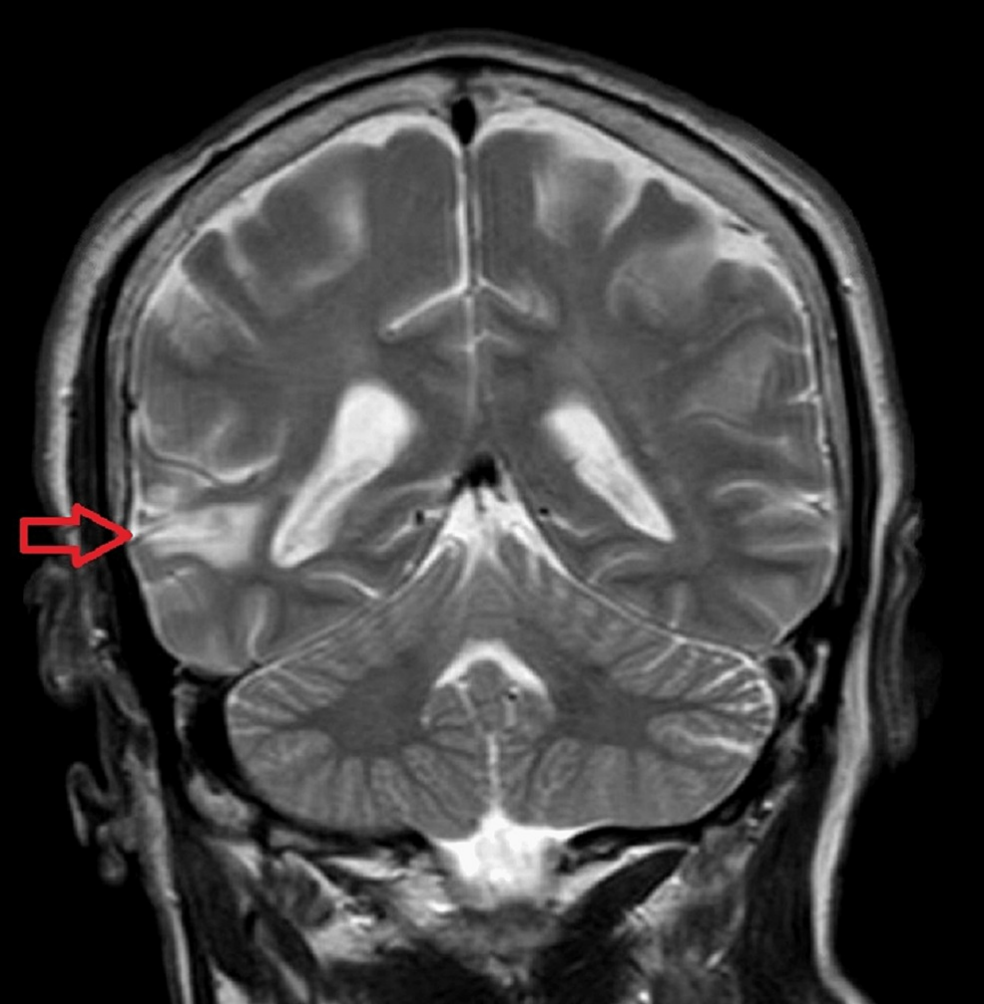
MRI, T2, coronal section, revealed encephalomalacia in the right temporal lobe (red arrow)
